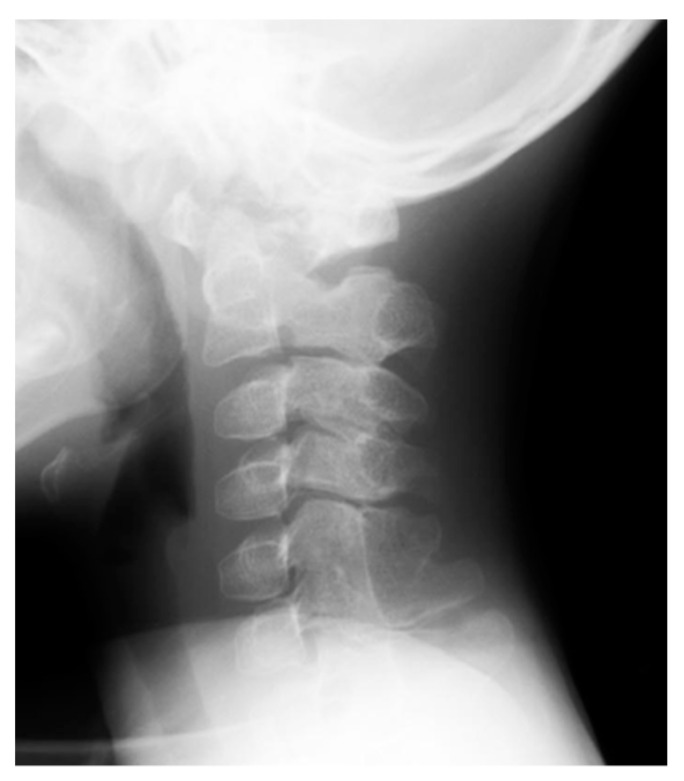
Lateral radiograph of the cervical spine of a FOP boy at the age of 7 years showing hypertrophy of the laminae and spinous processes and complete osseous fusions in facet joints and spinous processes between C5 and C6.
